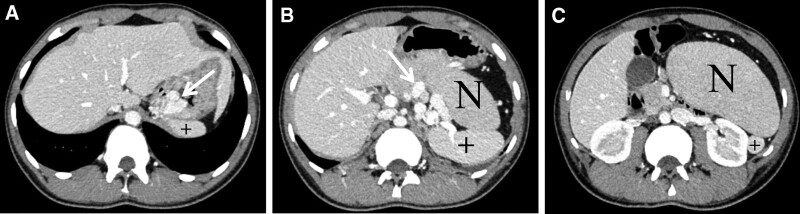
Dynamic contrast-enhanced CT scan findings (A–C). Solid arrow (→), varicose veins in the gastric fundus; asterisk (*), mass in the upper left abdomen; plus (+), normal spleen. CT = computed tomography.
